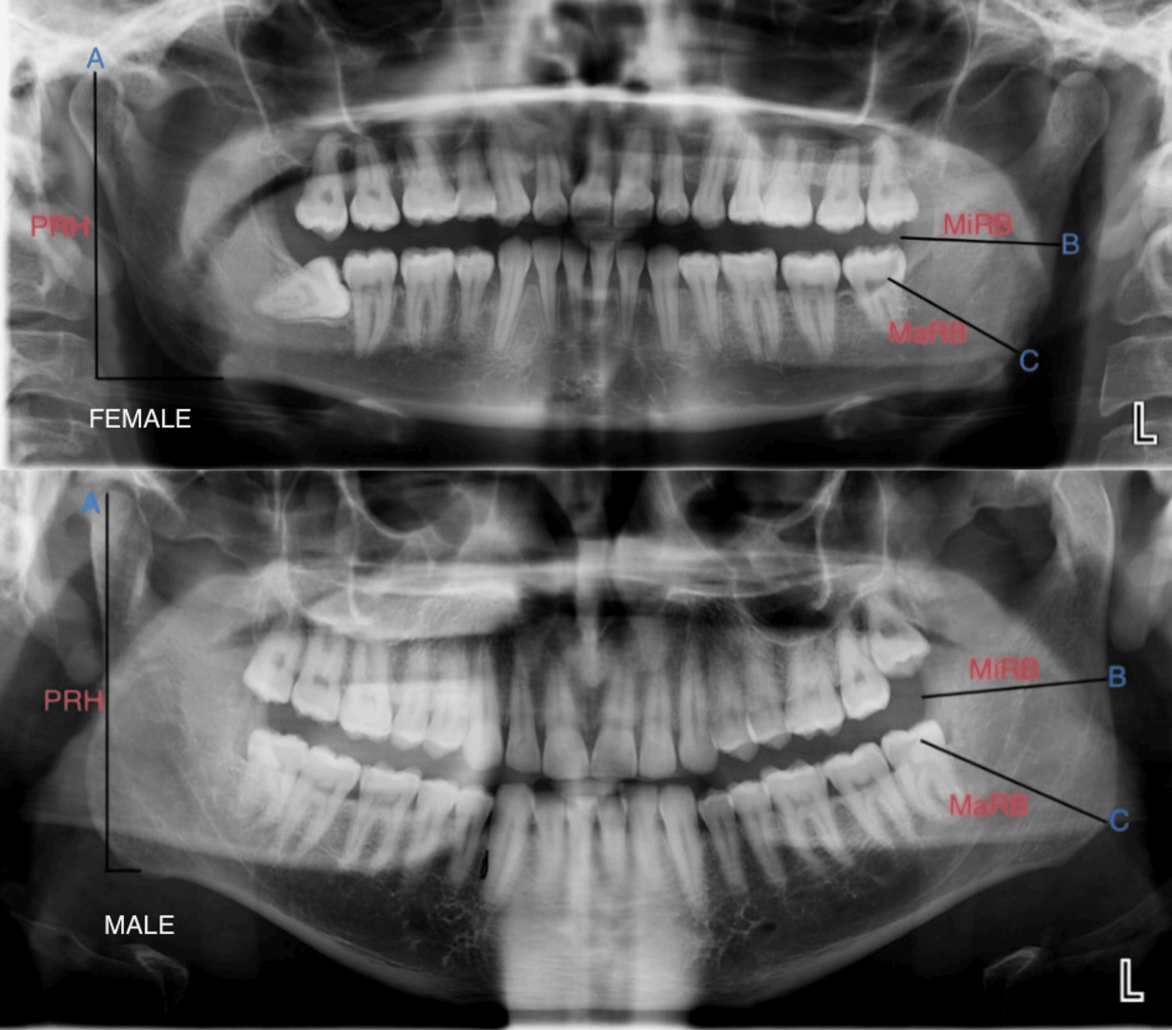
Representation of male and female orthopantomograms measuring various parameters like (A) projective ramus of the mandible (PRH), (B) minimum ramus breadth of the mandible (MRB), and (C) maximum ramus breadth (MaRB). The following parameters are projective ramus, which measures the distance between the highest point of the mandibular condyle and the lower margin of the bone; maximum ramus breadth, which measures the distance between the most anterior point on the mandibular ramus and a line connecting the most posterior point on the condyle and the angle of the jaw; and minimum ramus breadth, which measures the smallest anterior-posterior diameter of the ramus.
